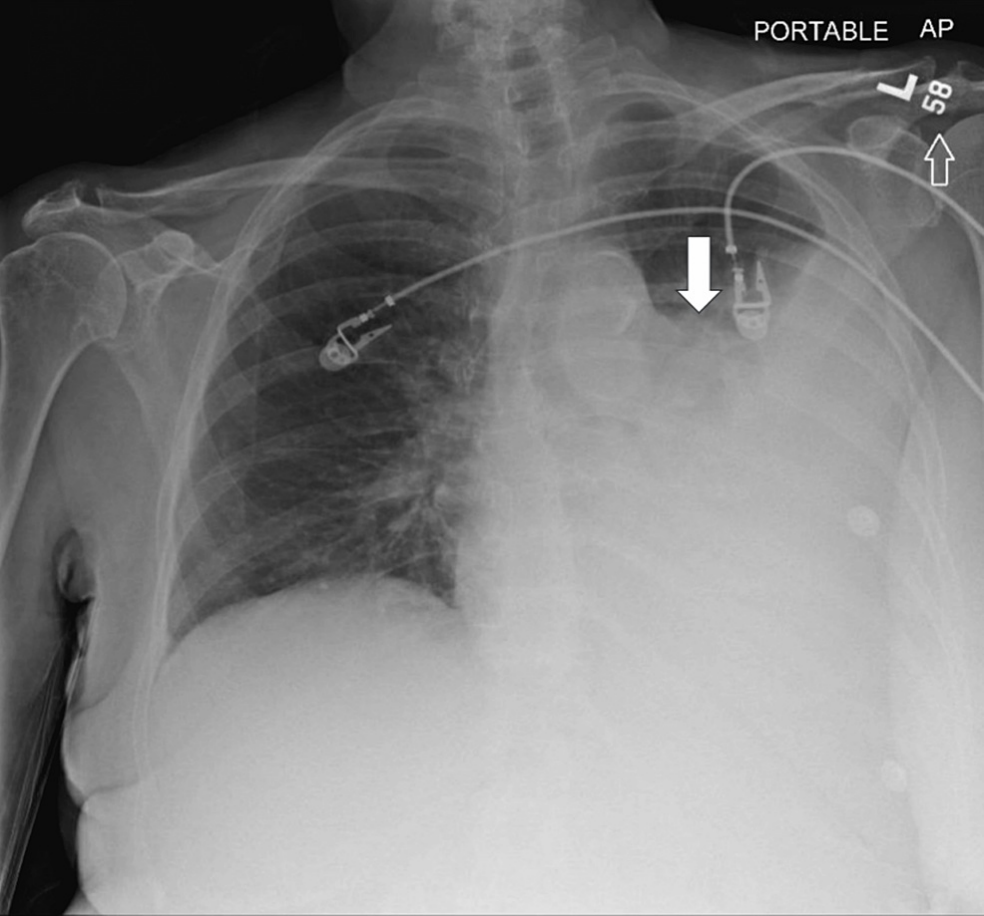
Chest x-ray of large pleural effusion (white arrow)
